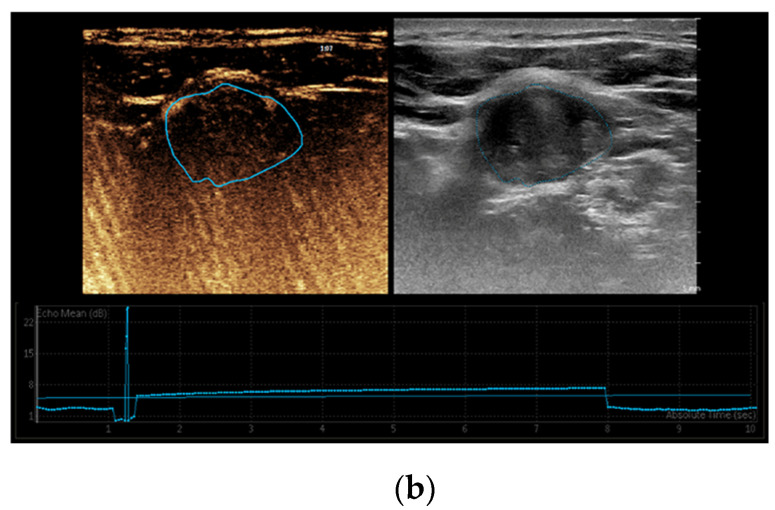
A 52 years old male with multifocal papillary thyroid carcinoma developed on multinodular goiter. (a) Quantitative shear wave elastography (SWE) revealed 38.8 kPa; (b) qualitative contrast-enhanced ultrasound (CEUS) of the nodule revealed heterogenous hypo-enhancement and quantitative CEUS revealed a time to peak of 10.07 s and a peak intensity of 3.18 dB.
